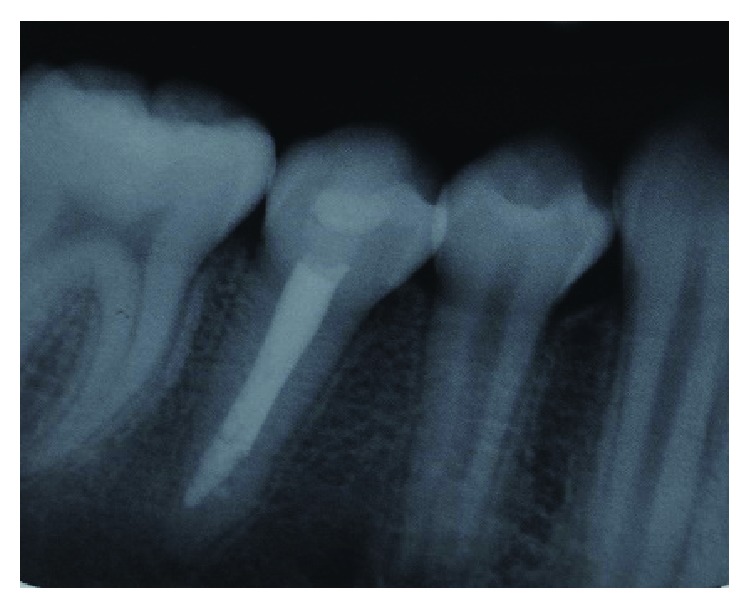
Periapical radiograph of tooth 45, 6-month postapexification showing complete root maturogenesis.
